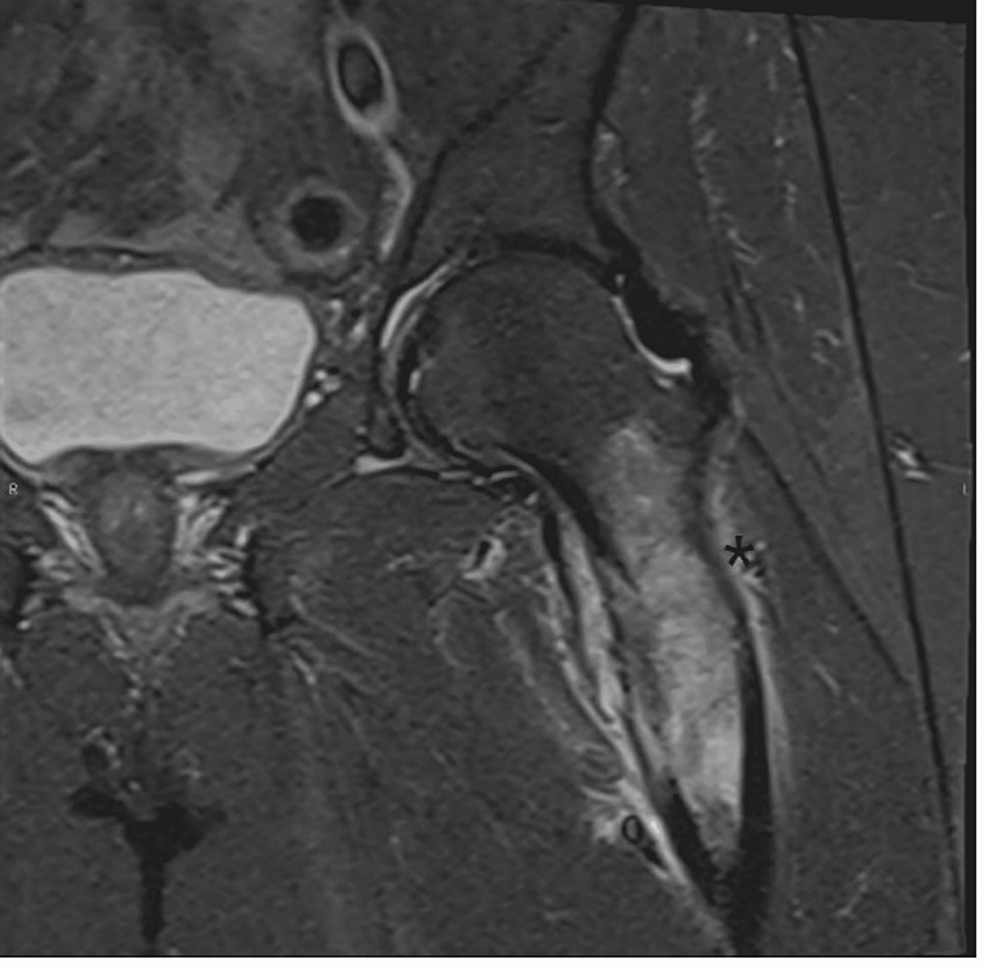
Coronal MRI of the pelvis, STIR sequence, demonstrating a destructive and infiltrating lesion in the left proximal femur (*) with periosteal reaction and bone edema. STIR, short tau inversion recovery
